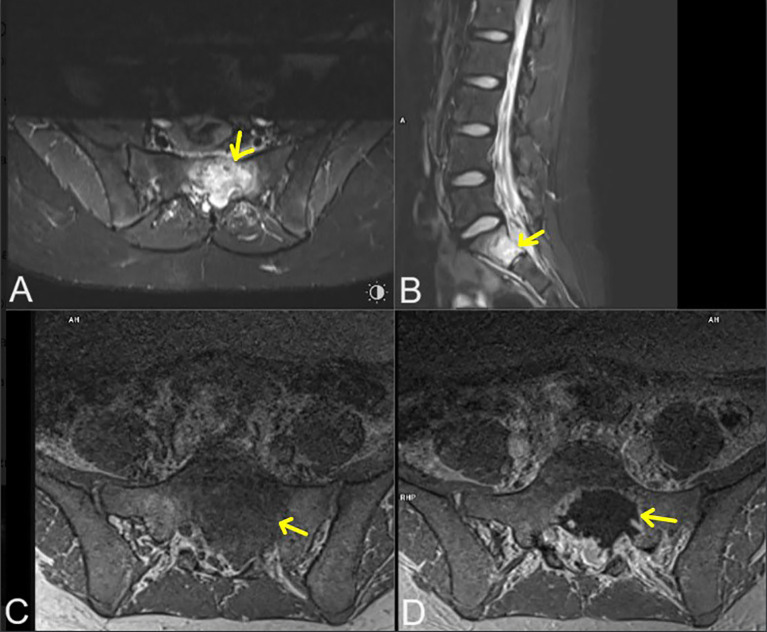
Lumbosacral magnetic resonance imaging in: T2-weighted (STIR) axial projection (A), T2-weighted (STIR) sagittal (B), T1-weighted (vibe) axial (C), T1-weighted (vibe) axial with contrast agent administration (D). Metastatic lesions marked with yellow arrows.
